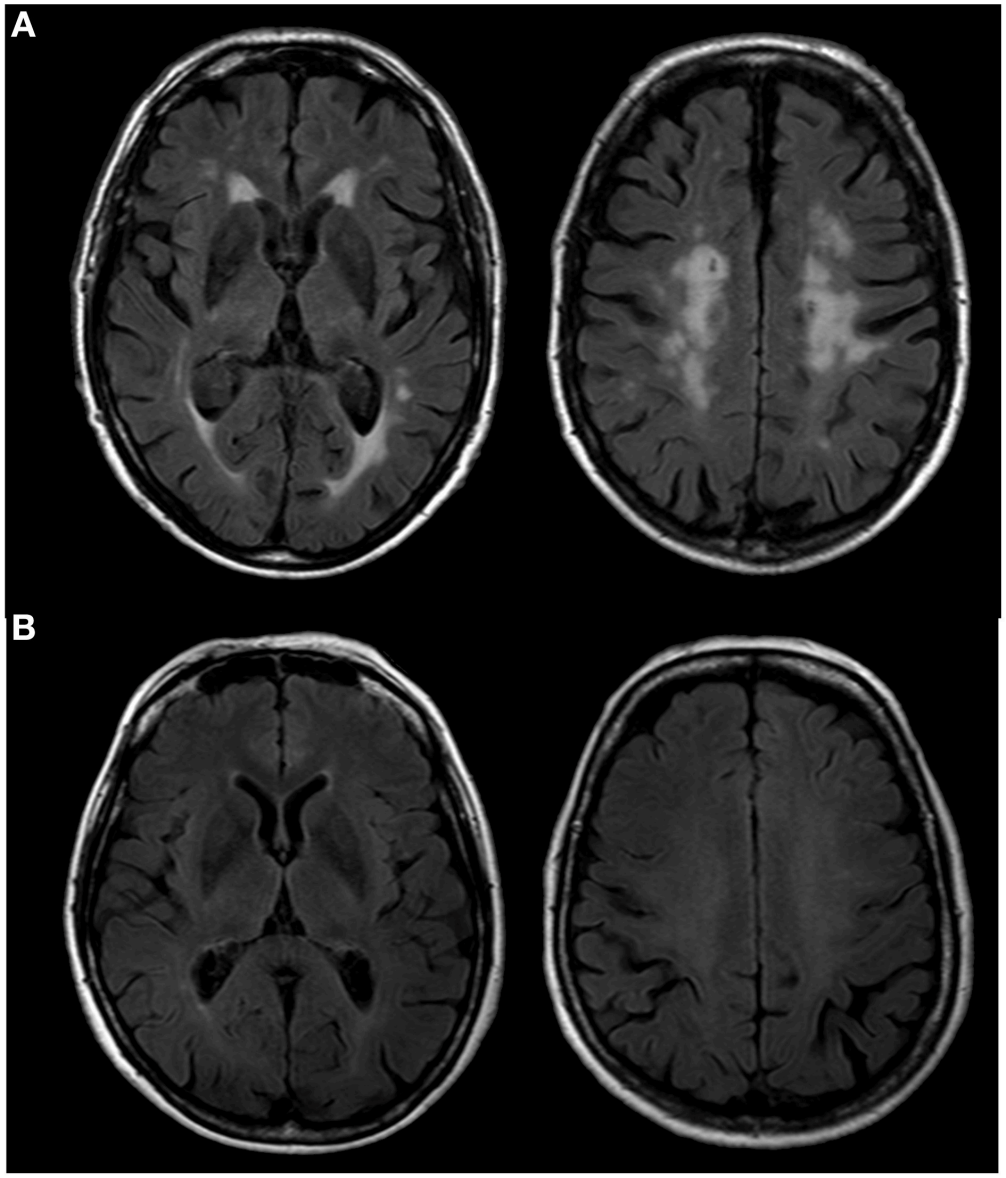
FLAIR scans of older subjects presenting a high (A) and a low (B) level of WMH according to the Fazekas scale.
